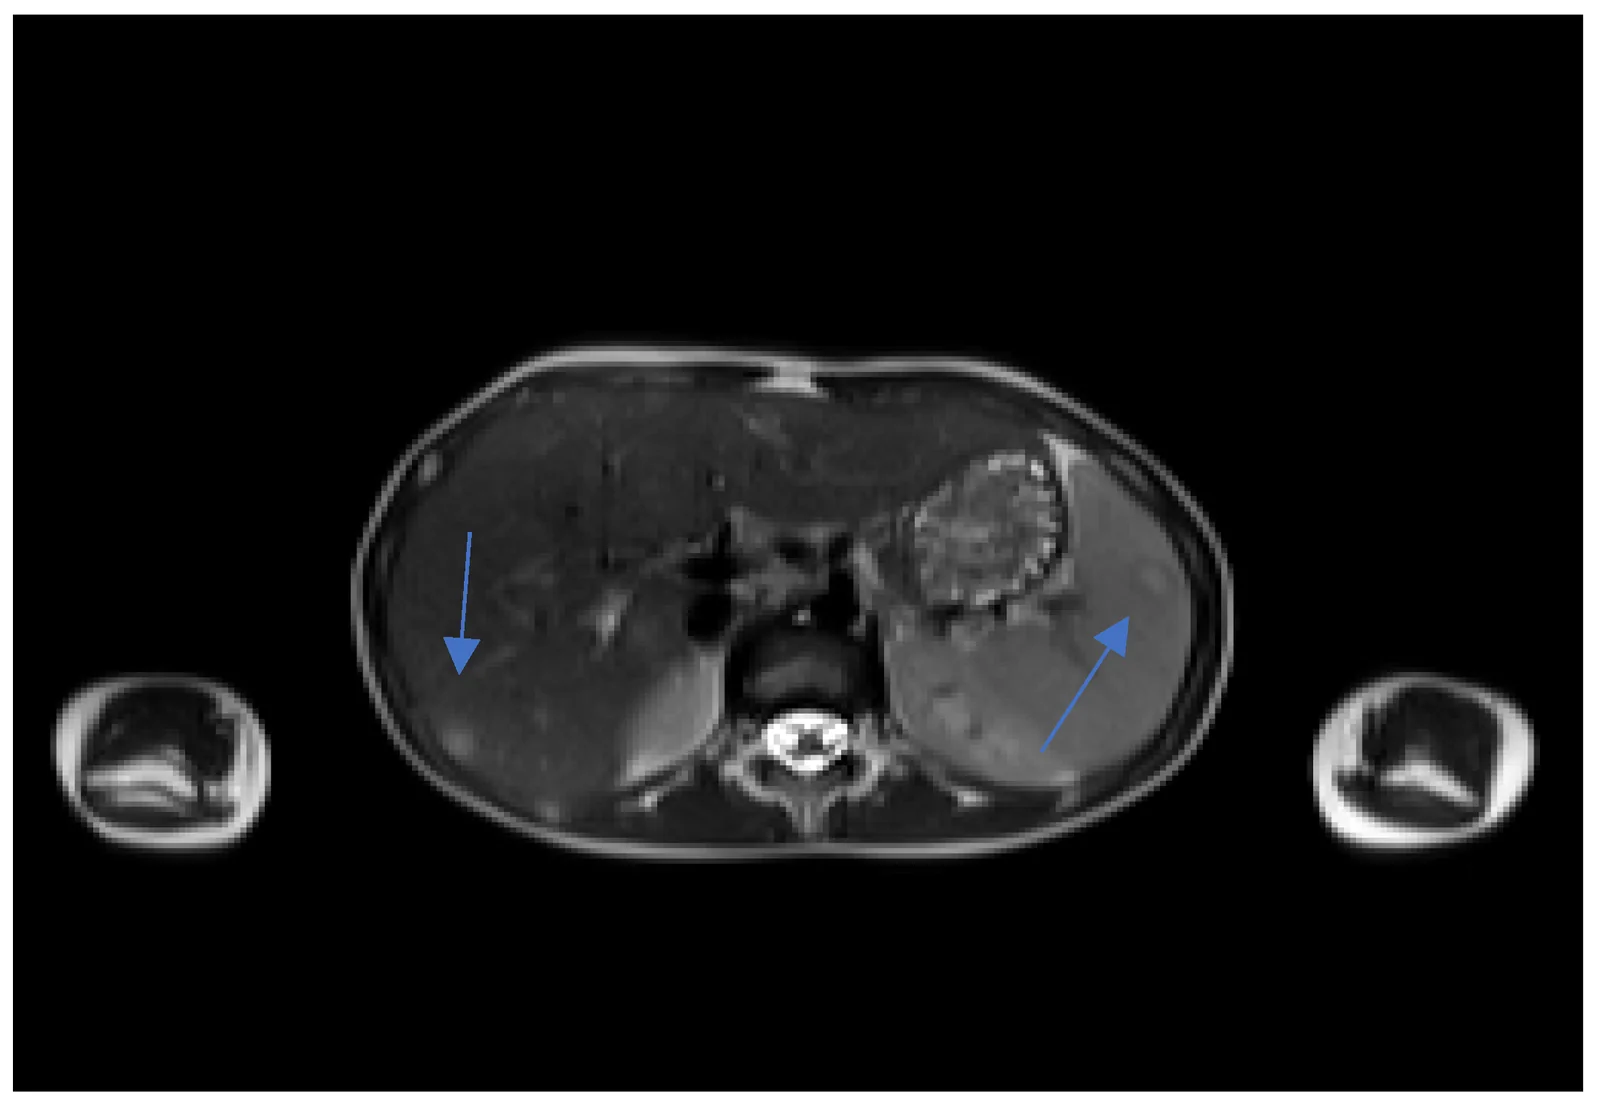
Axial T2-weighted MRI image showing focal hepatic and splenic lesions (arrows).
